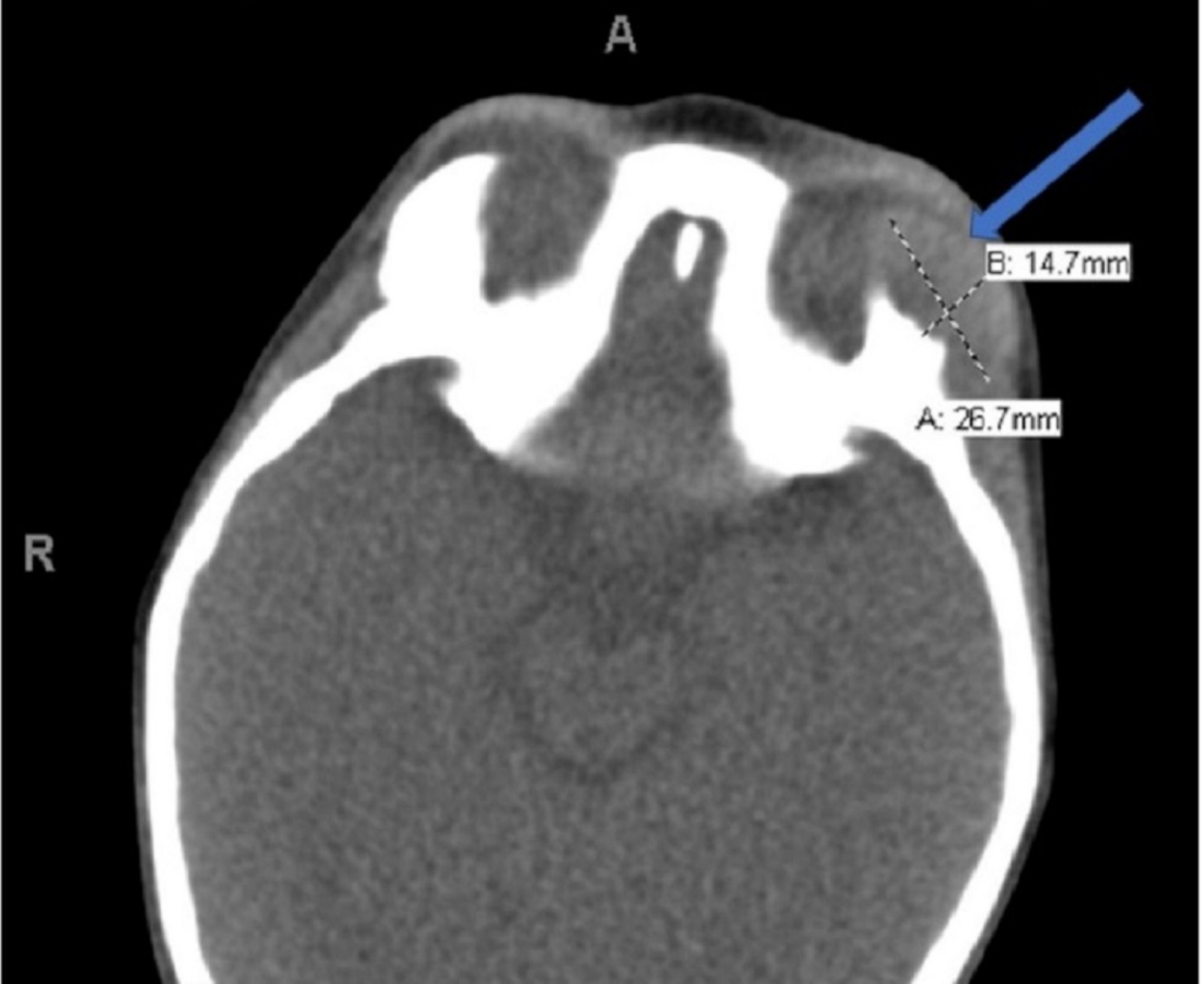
CT of lytic lesion concerning for eosinophilic granuloma (EG) or hematologic malignancy (HM). Sharply defined lytic lesion zygomatic process of the left frontal bone forming the superolateral wall of the left orbit with intraorbital extension; also extension to the left temporal fossa as described. Findings probably indicate eosinophilic granuloma. Differential is hematologic malignancy; mild left proptosis; hematologic workup requested for further assessment; biopsy of the lesion with imaging guidance can be considered following metastatic and hematologic workup.
